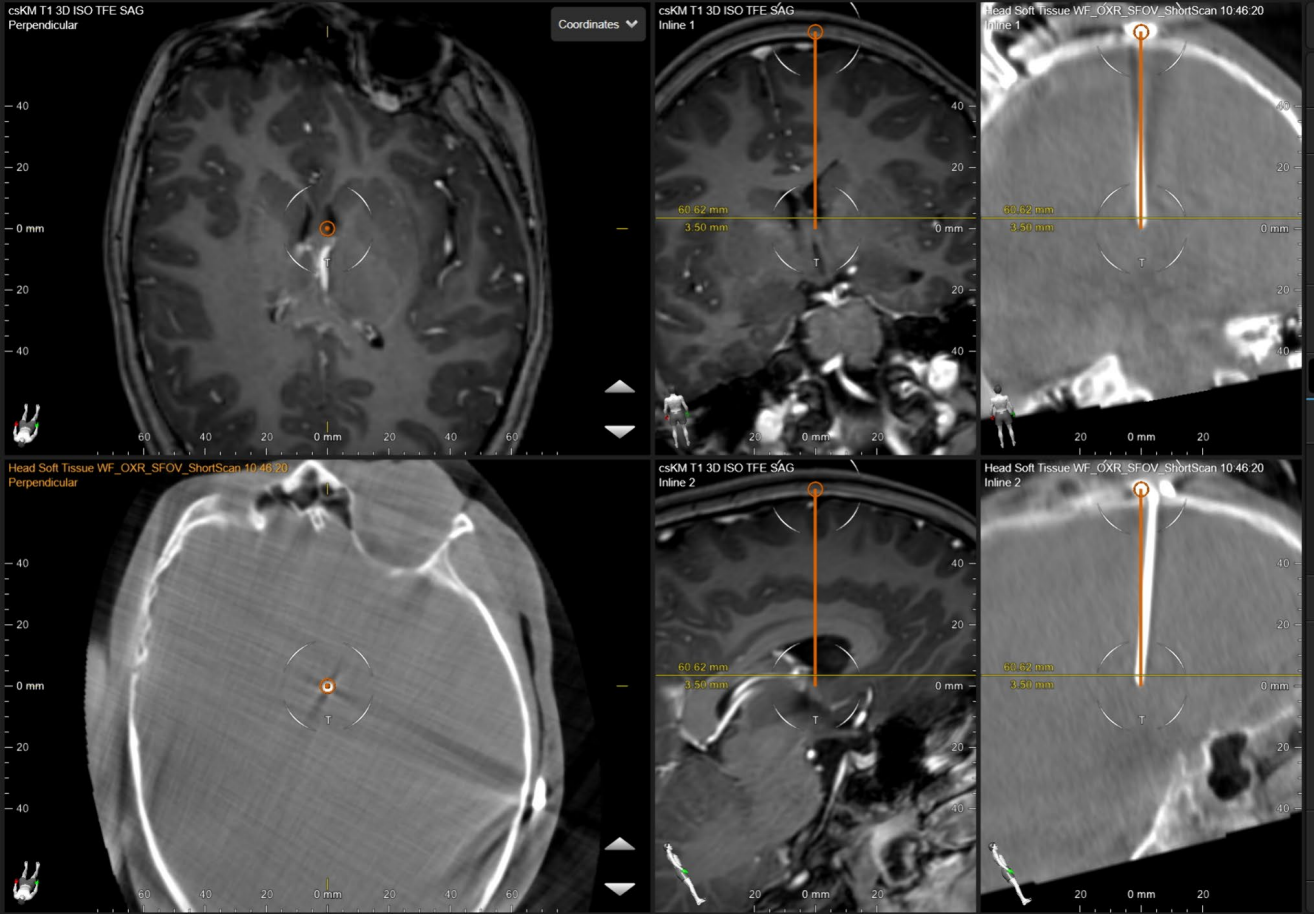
Intraoperative screenshot of the navigation system. The intraoperatively acquired images of the cone-beam-CT have been merged with the preoperative MRI within the neuronavigation system. The planned trajectory of the ventricular catheter is shown in orange
